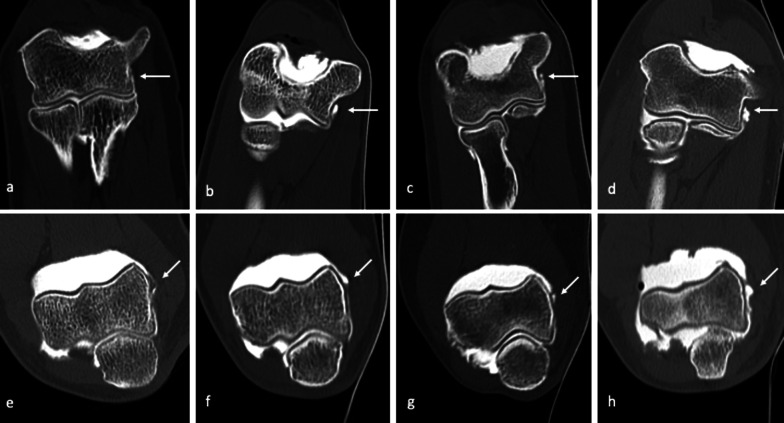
CT-arthrography coronal images (a–d) and axial images (e–h) belonging to four different patients, three of which display different aspects of ligament derangement involving the A-MCL (white arrows). Intact anterior band (A-MCL) of the medial collateral ligament (MCL) complex (a, e and b, f). Widening of the medial aspect of the articular recess, associated to a “bow-shaped” loose A-MCL, with no evidence of fiber tearing, suggestive of ligament laxity (b, f). Thickened and irregular A-MCL, showing a partial-thickness tear (c, g) and a full-thickness tear (d, h); contrast agent progressively spills through the ligament, as fibers are gradually disrupted as a consequence of micro-tearing and damage progresses from a partial to a complete tear
